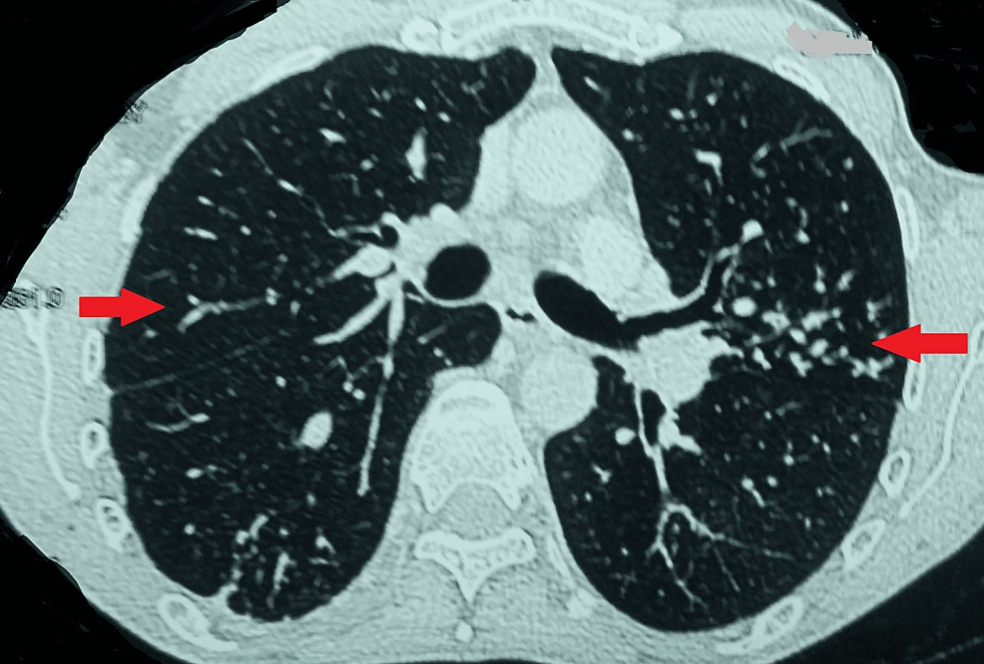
A follow-up contrast-enhanced computed tomography of the chest suggestive of acute-on-chronic infective sequelae
